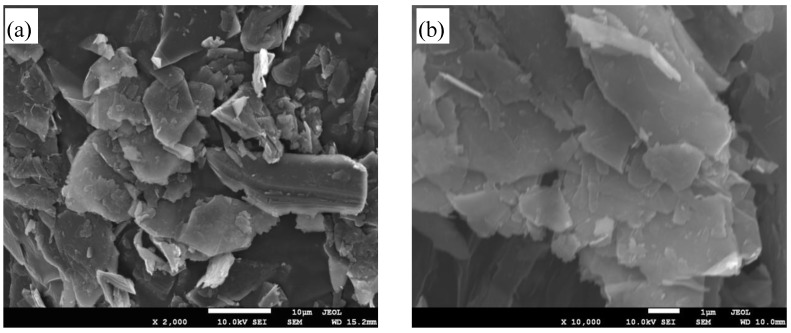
SEM images of (a) graphite prepared from nature graphite by ultrasonication for 30 min and (b) exploited graphite (EG).
